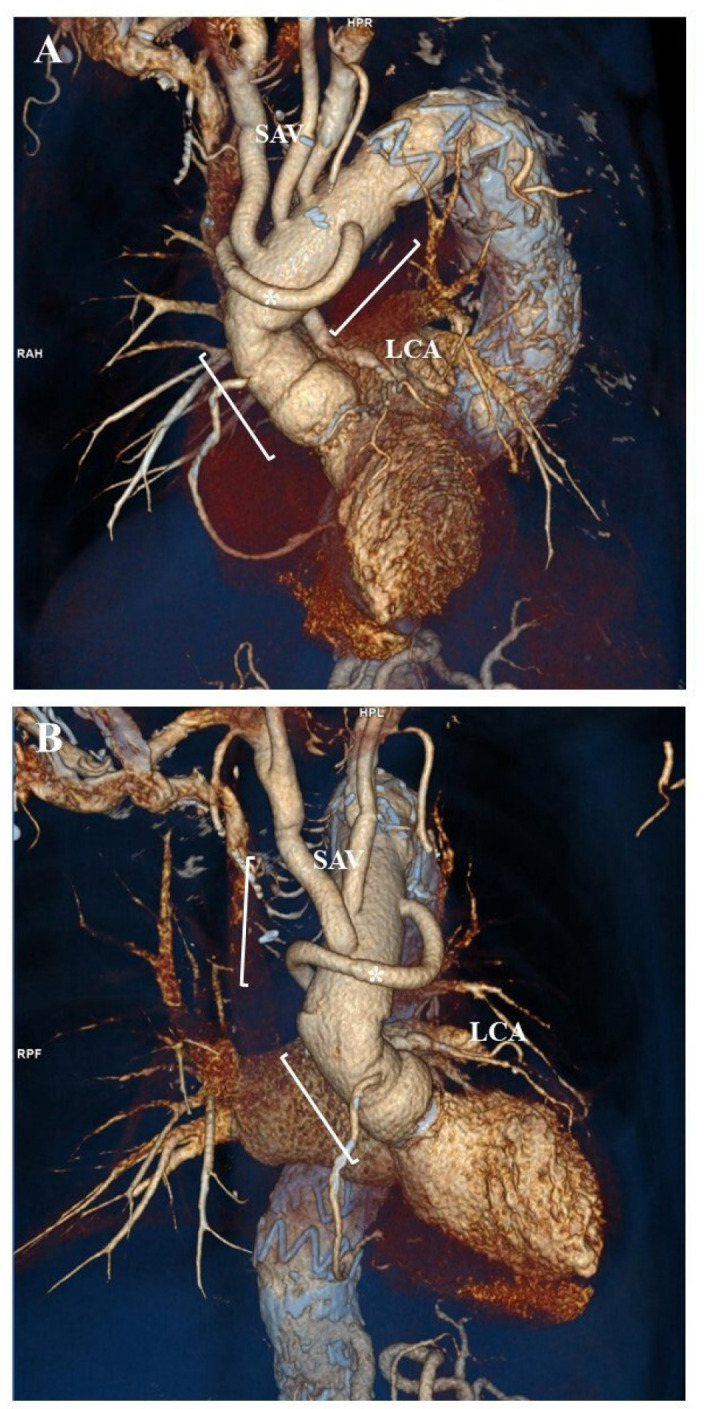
Volume-rendering computed tomography angiography after surgery showing
